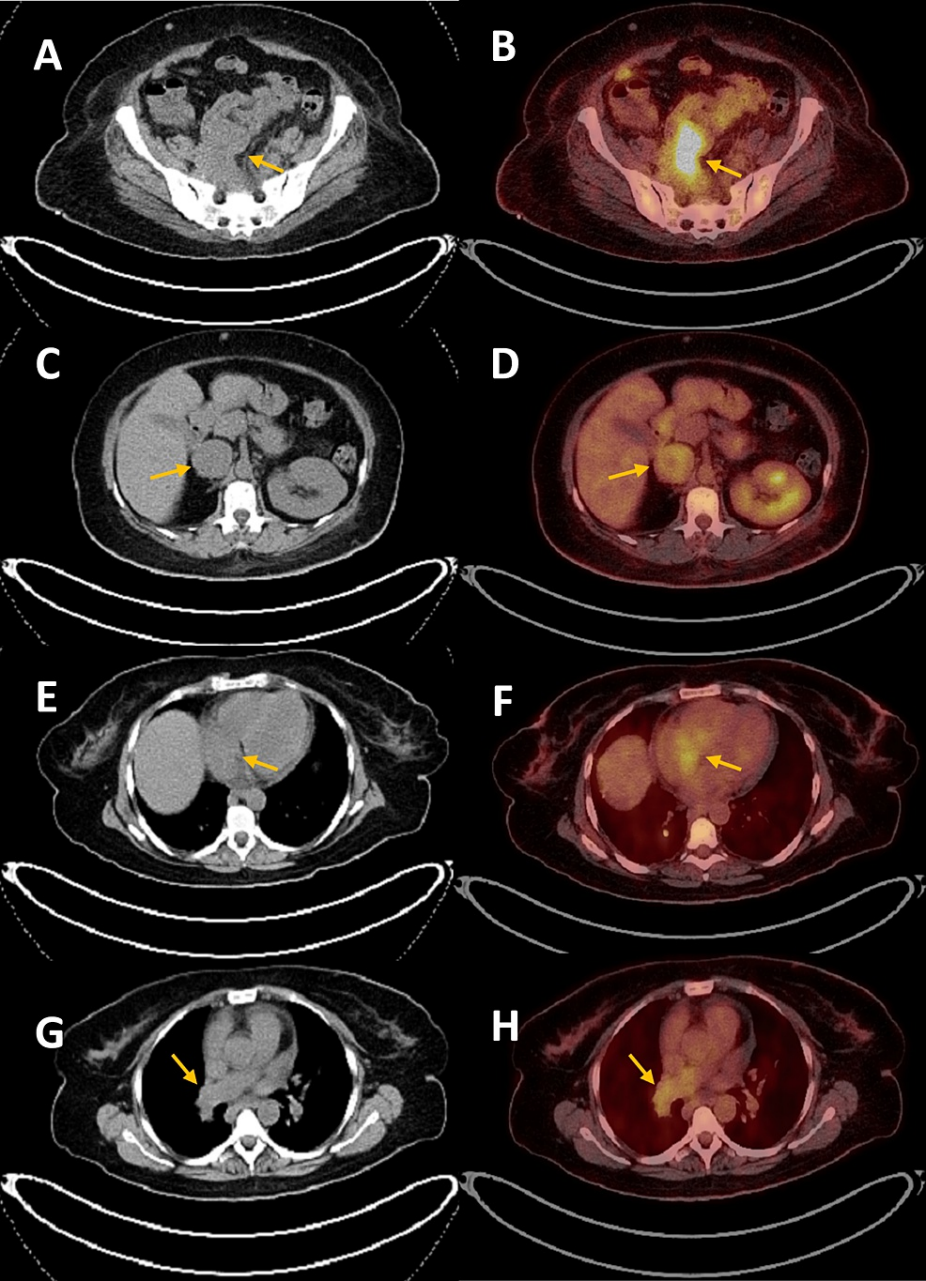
Axial 18F-FDG PET/CT without contrast of the whole body. Scans (A,B) reveal increased FDG activity of the irregularly shaped soft tissue mass (orange arrows in A,B) invading the right psoas muscle, which is inseparable from the adjacent small bowel loops at the pelvic inlet. The dimensions of the mass are 6.0 cm x 3.1 cm (SUVmax 8.3), which previously was measured at 3.6 cm x 2.6 cm (SUVmax 2.5). Also, it shows (C,D) inferior vena cava enlargement (orange arrows in C,D) with increased FDG activity, (E,F) high FDG uptake of the right atrial mass (orange arrows in E,F), and (G,H) high FDG uptake of the right pulmonary embolism (orange arrows in G,H). 18F-FDG PET/CT: Flourine-18 fluorodeoxyglucose positron emission tomography/computed tomography
